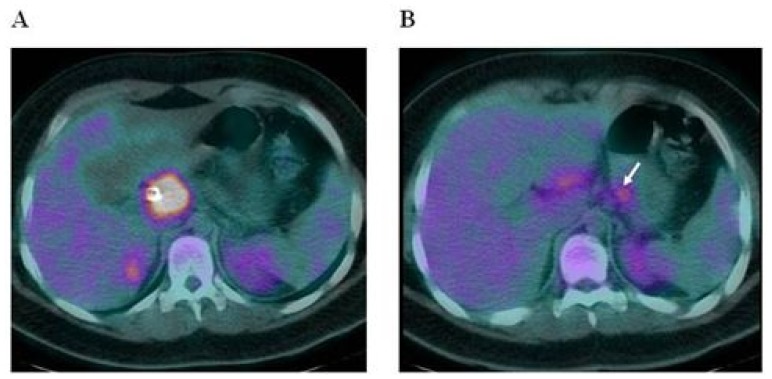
^18^F-FDG PET/CT showed increased glucose metabolism in a larger lesion in the head of pancreas (Figure 1A), as well as in one more smaller area in the tail of the pancreas (pointed by the arrow) and in one lymph node in the hepato-gastric ligament (Figure 1B).
